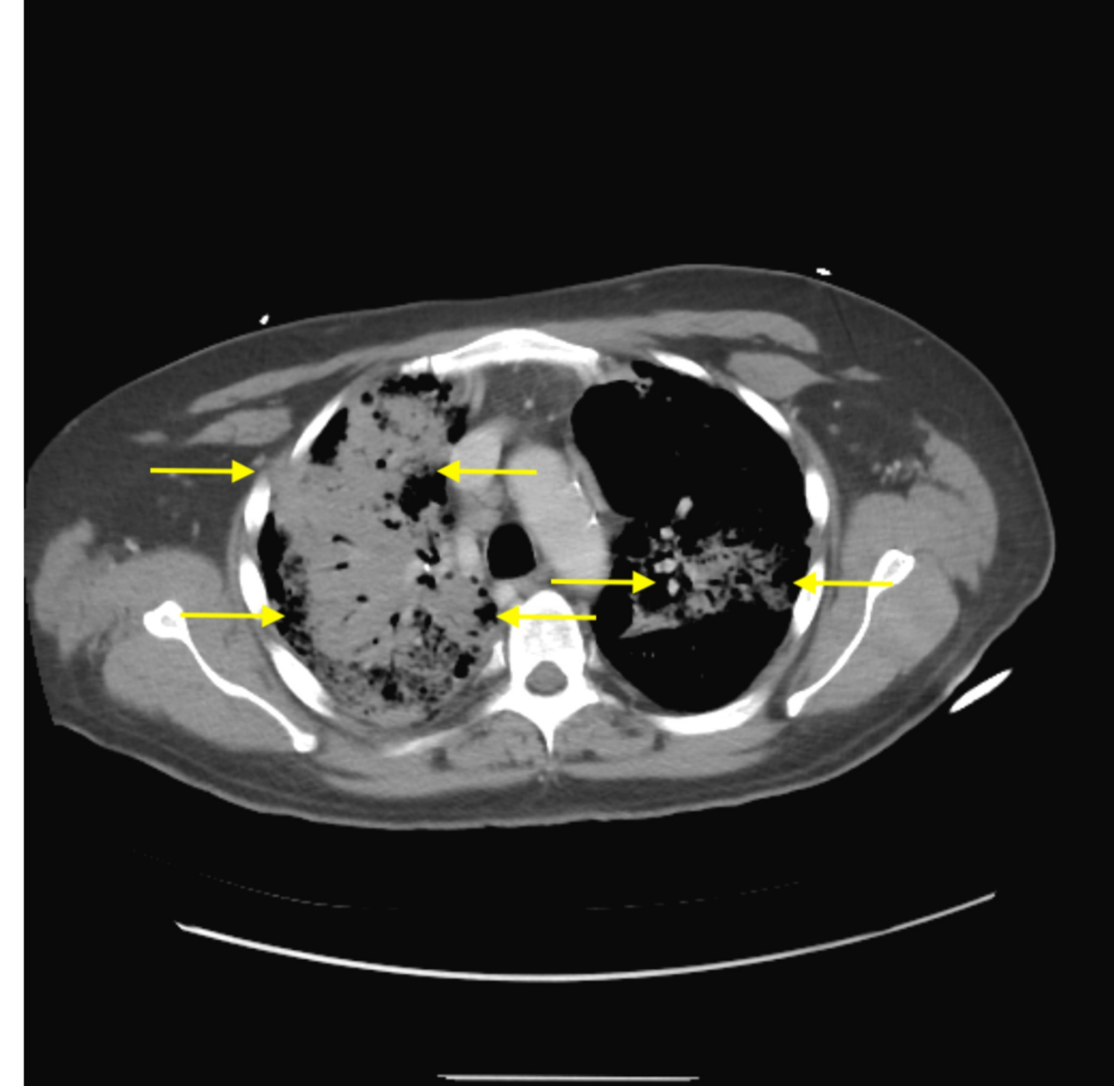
CT chest without contrast The image displays dense airspace consolidations (yellow arrows); BAL obtained shortly after radiography revealed diffuse alveolar hemorrhage CT: computed tomography; BAL: bronchoalveolar lavage
